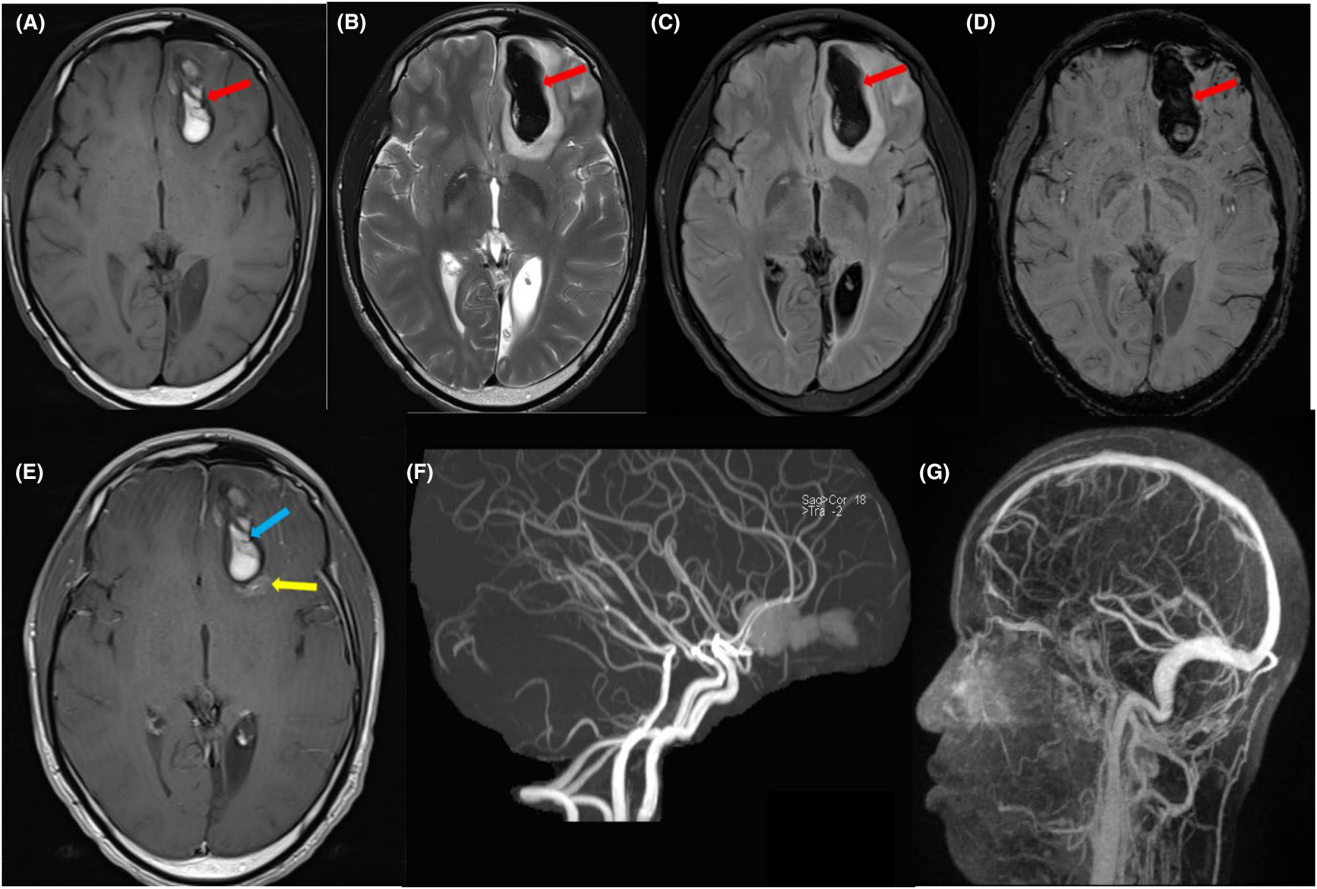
MRI head with contrast (A) T1, (B) T2, (C) FLAIR, (D) SWI, and (E) T1 post contrast shows the left frontal parasagittal hematoma with high signal intensity on T1, low signal in T2 and FLAIR, and significant SWI blooming (red arrows). Mild surrounding edema is causing a mass effect. There is no pathological post‐contrast enhancement suggesting tumor (blue arrow); however, there is a contrast blush posterior to the hematoma (yellow arrow). (F) Intracranial MRA is unremarkable with no major vascular occlusion, stenosis, aneurysm, or AVM. (G) MRV appears unremarkable with no filling defects.
